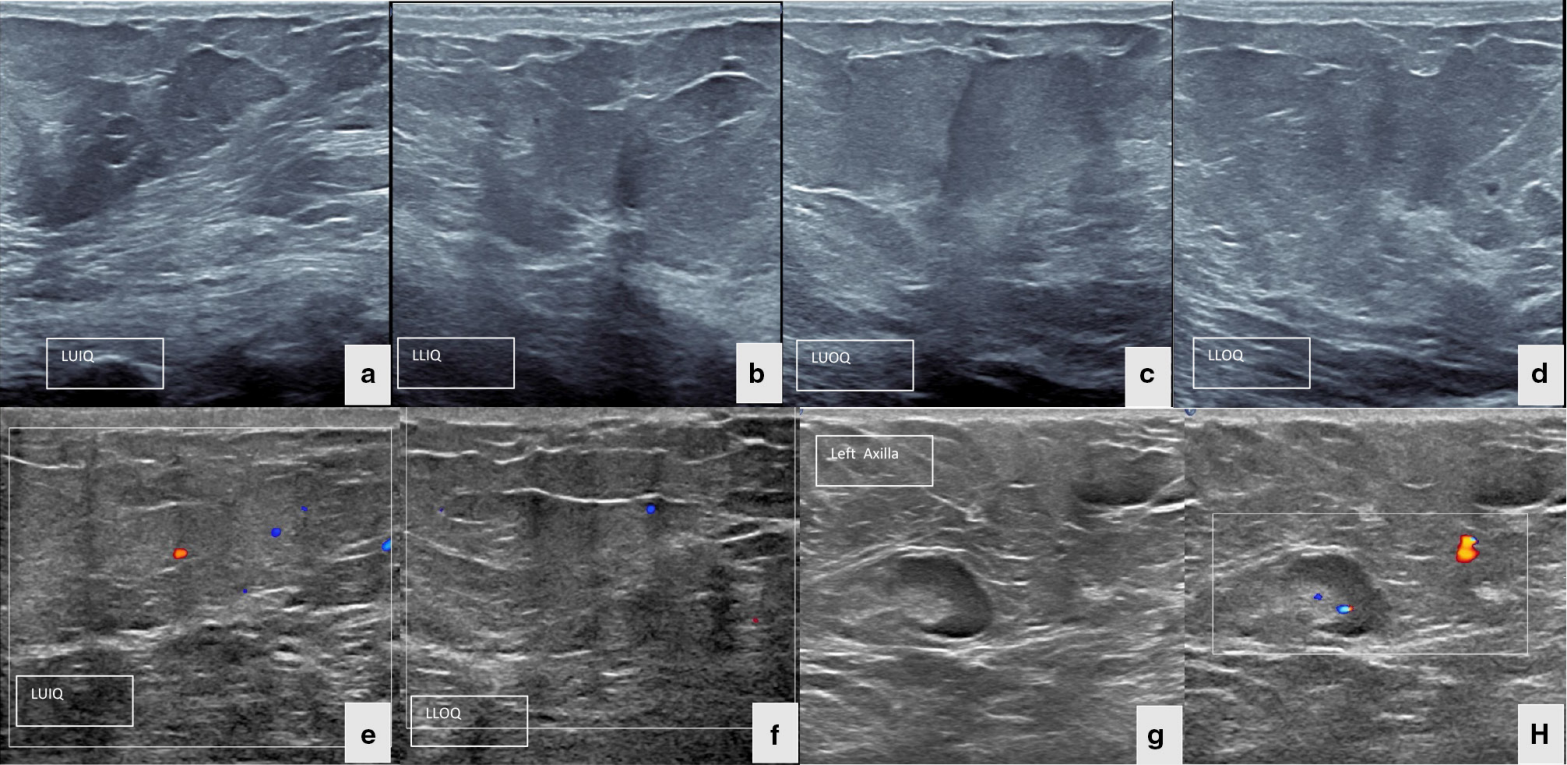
(a–h) Ultrasound and colour doppler of the four quadrants of the left breast demonstrate normal parenchyma and blood flow.
